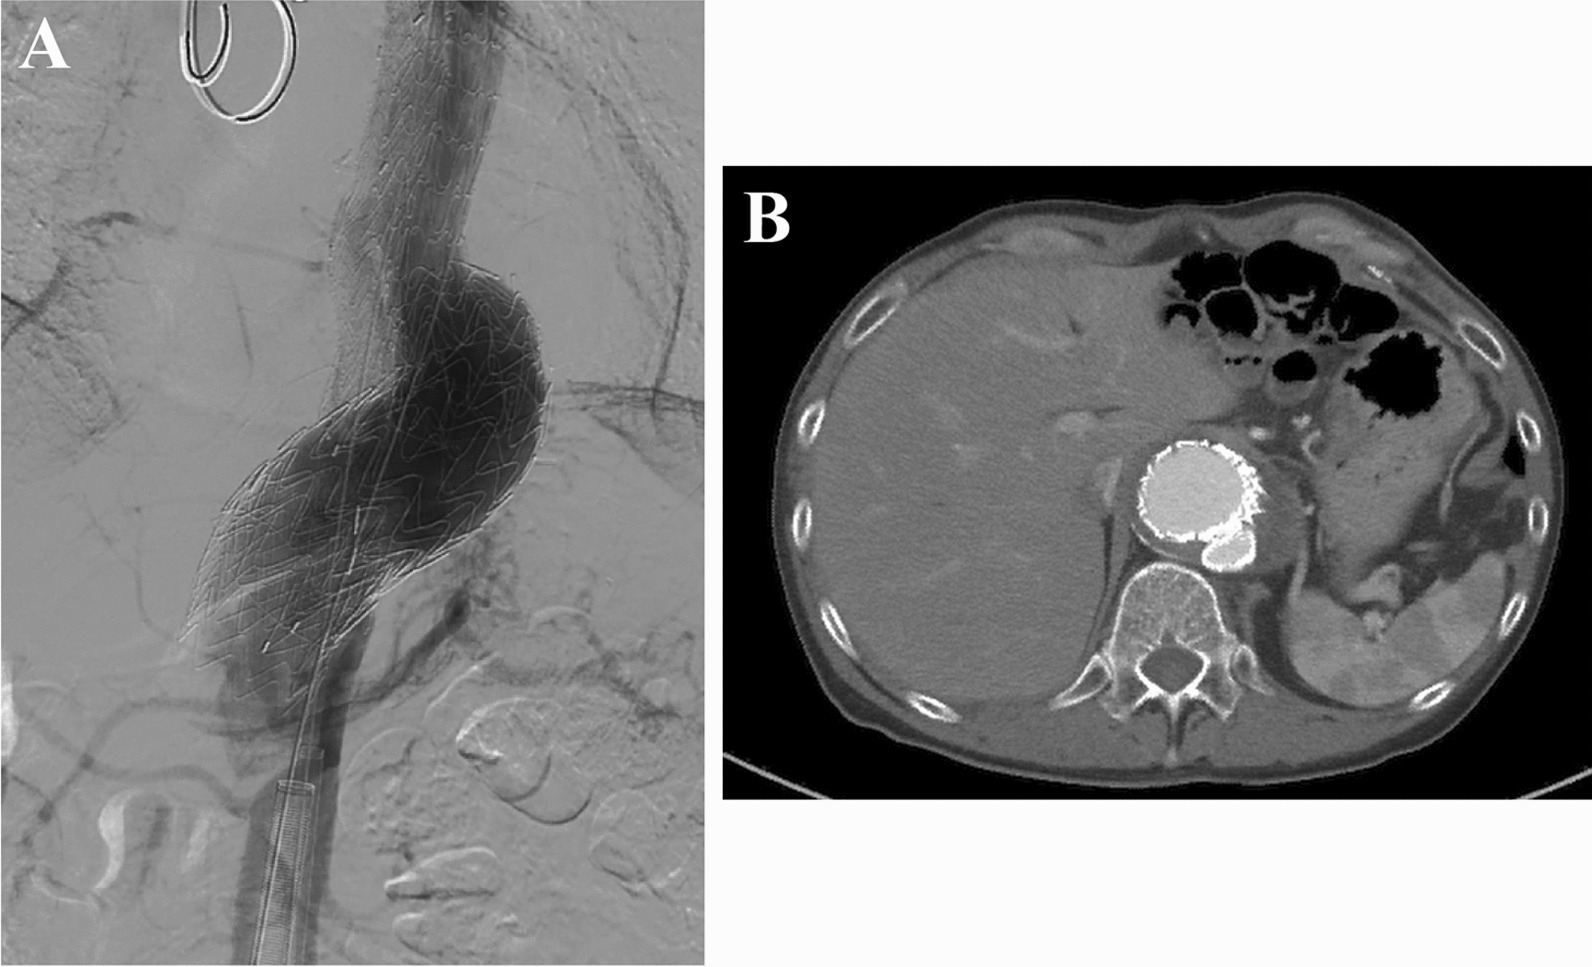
Intra- and postoperative findings. A Intraoperative findings under digital subtraction angiography. B Postoperative contrast-enhanced computed tomography scan
